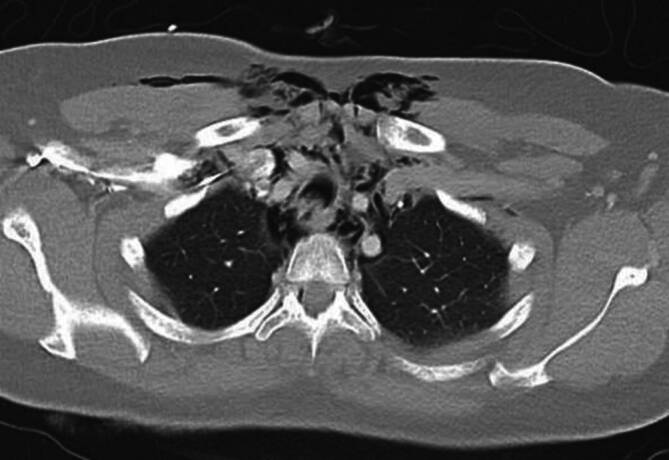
Thorax-CT bei Schockraumaufnahme: Nachweis eines ausgeprägten Weichteilemphysems mit begleitendem Pneumomediastinum
